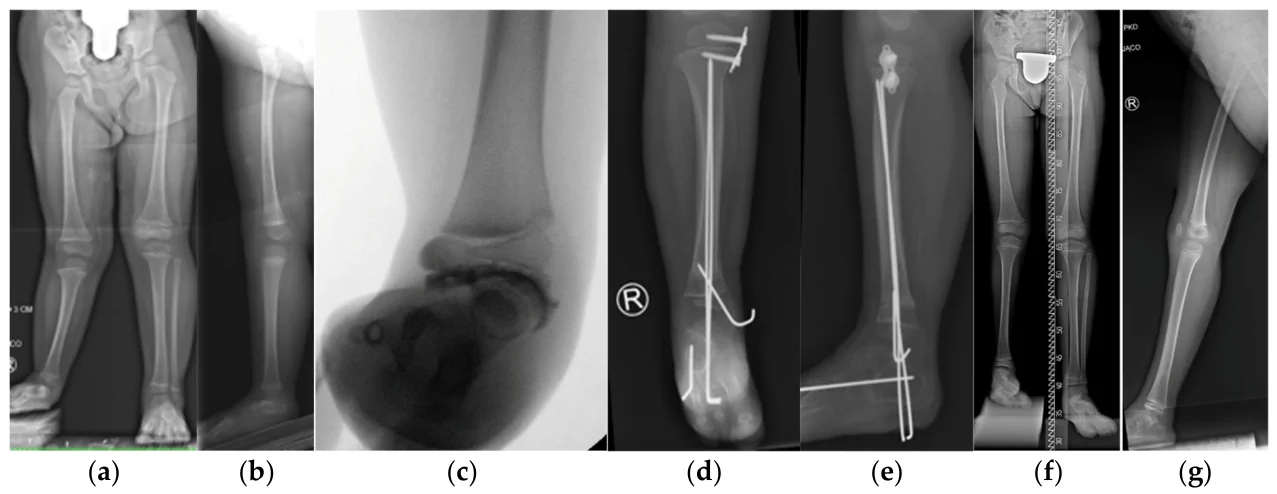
Radiographic evaluation of type 3C FH: (a,b) preoperative radiograph; (c) ankle arthrography; (d,e) radiograph obtained 6 weeks after SUPERankle procedure; (f,g) radiograph obtained 1 year postoperatively. R—right lower limb, NA STOJĄCO—standing position.
